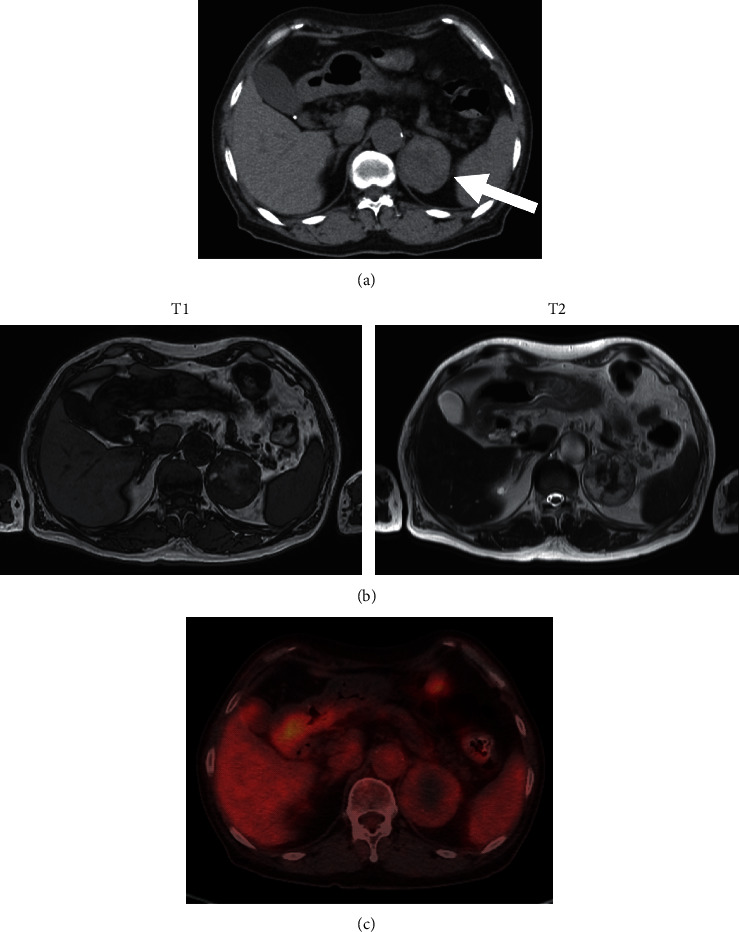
(a) Abdominal plain CT. Arrow: left adrenal mass (52 mm × 50 mm). (b) T1-weighed (left) and T2-weighed (right) images of plain MRI. (c) PET-CT.
